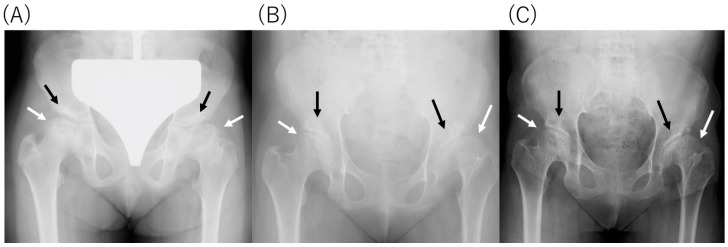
X-ray of the pelvis (A) pelvis at 9 years old, 3 years before HSCT, (B) pelvis at 15 years old, 3 years post-HSCT, (C) pelvis at 29 years old, 17 years post-HSCT. Dysplasia of the hip acetabulum (black arrow) and deformation of the femoral head (white arrow) are observed. No obvious narrowing of the joint space has been seen.
